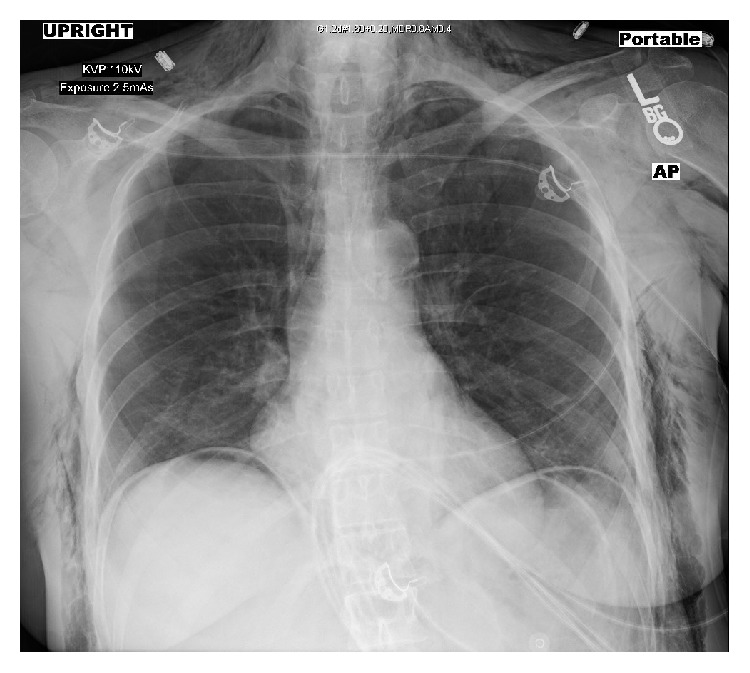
Chest X-ray showing significant subcutaneous emphysema of lower neck and bilateral chest walls, significant free intraperitoneal air, and small bilateral pneumothoraces. The emphysema was caused by laparoscopic robotic myectomy.
